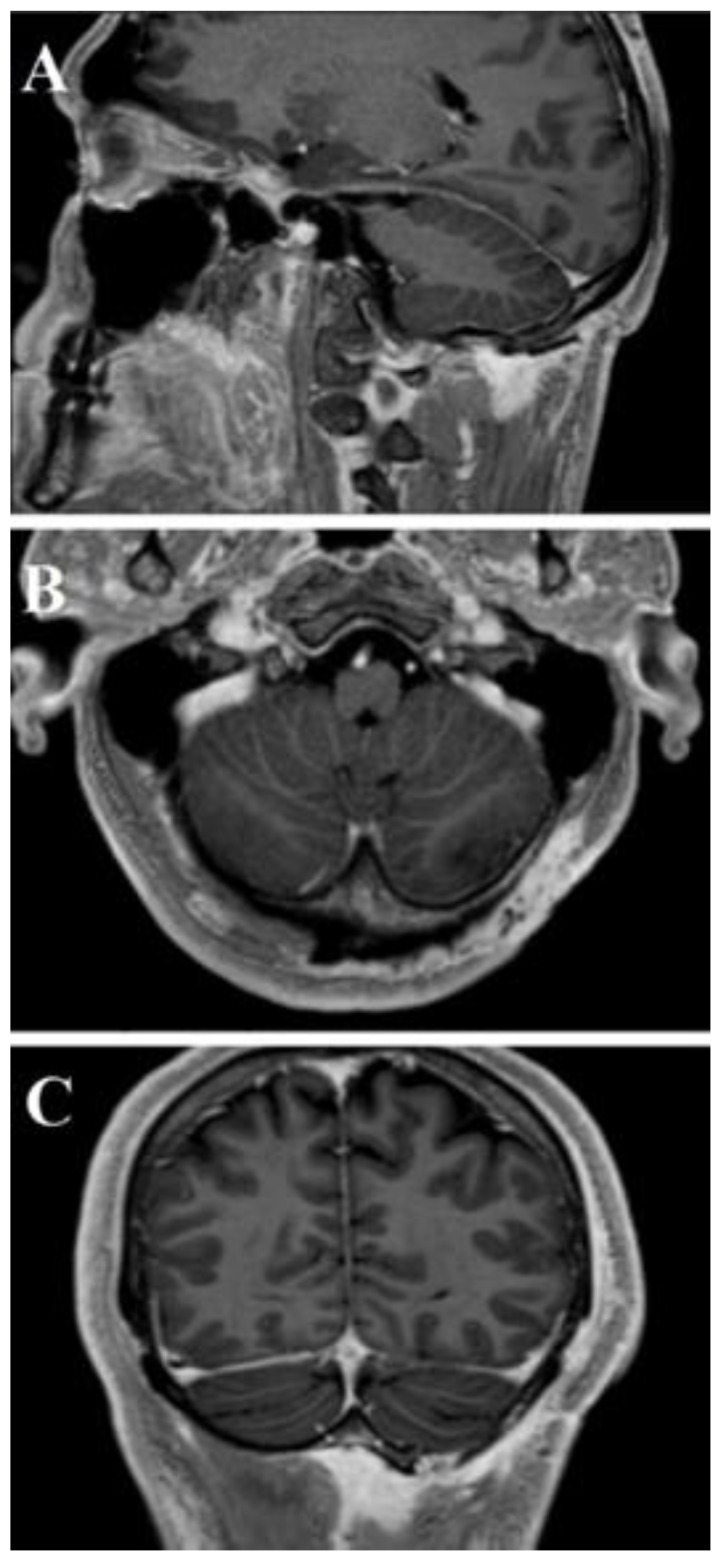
Post-operative head and neck MRI T1-WI with Gadolinium—sagittal (A), axial (B), and coronal (C) areas showed absence of residual tumor.
